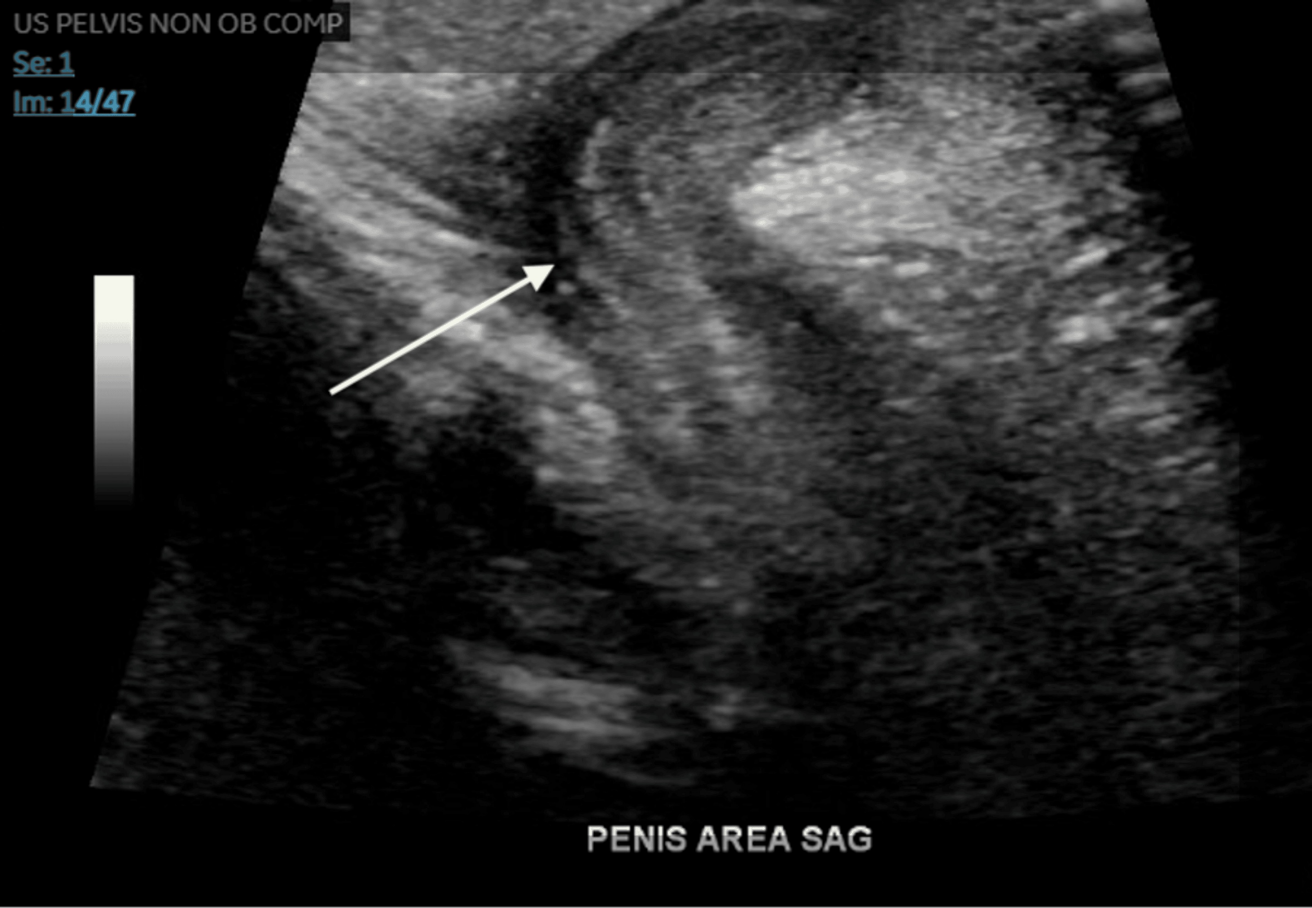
Pelvic ultrasound demonstrating a microphallus
